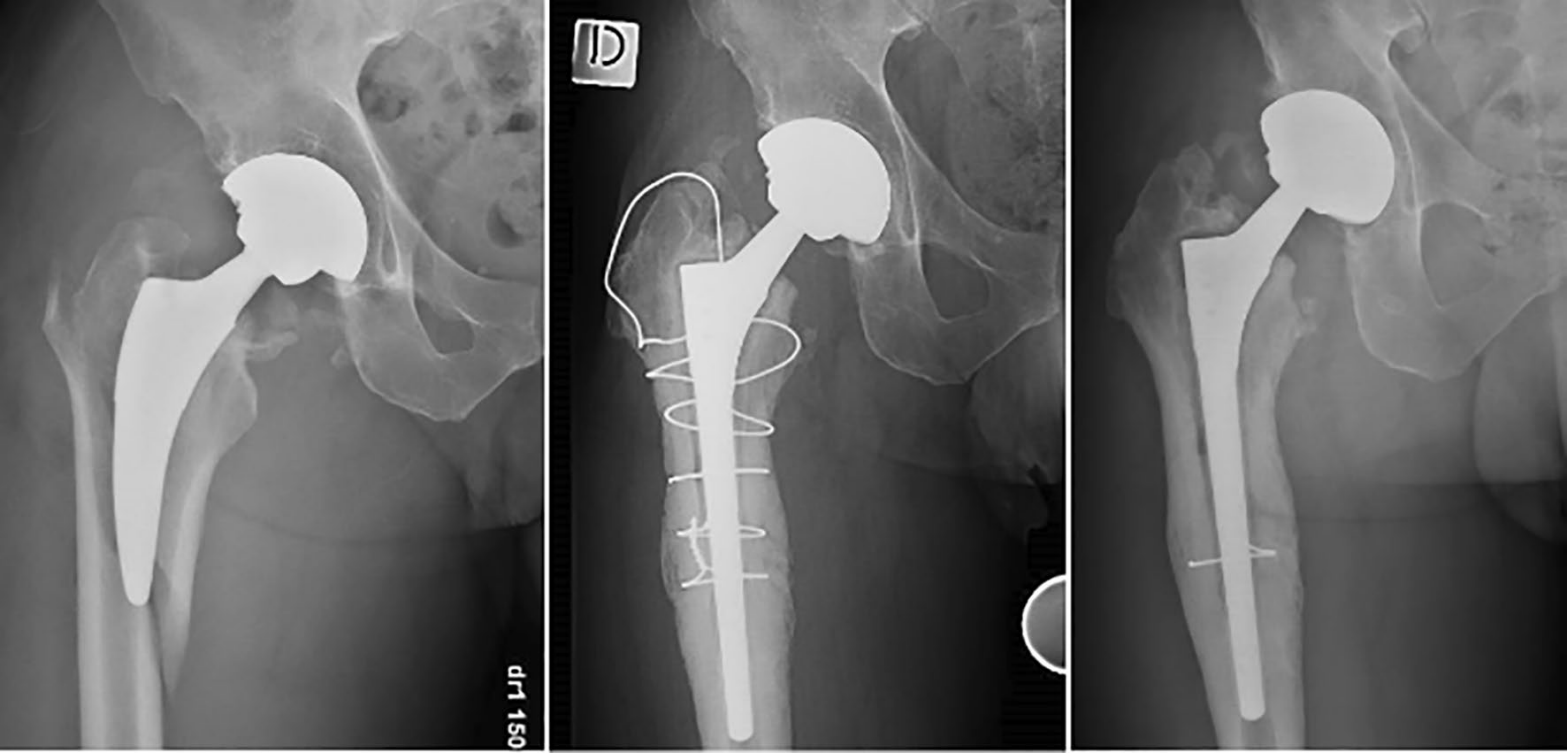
Radiographic analysis of a sample case with final cerclage removal performed after 21 months from indexed surgery
